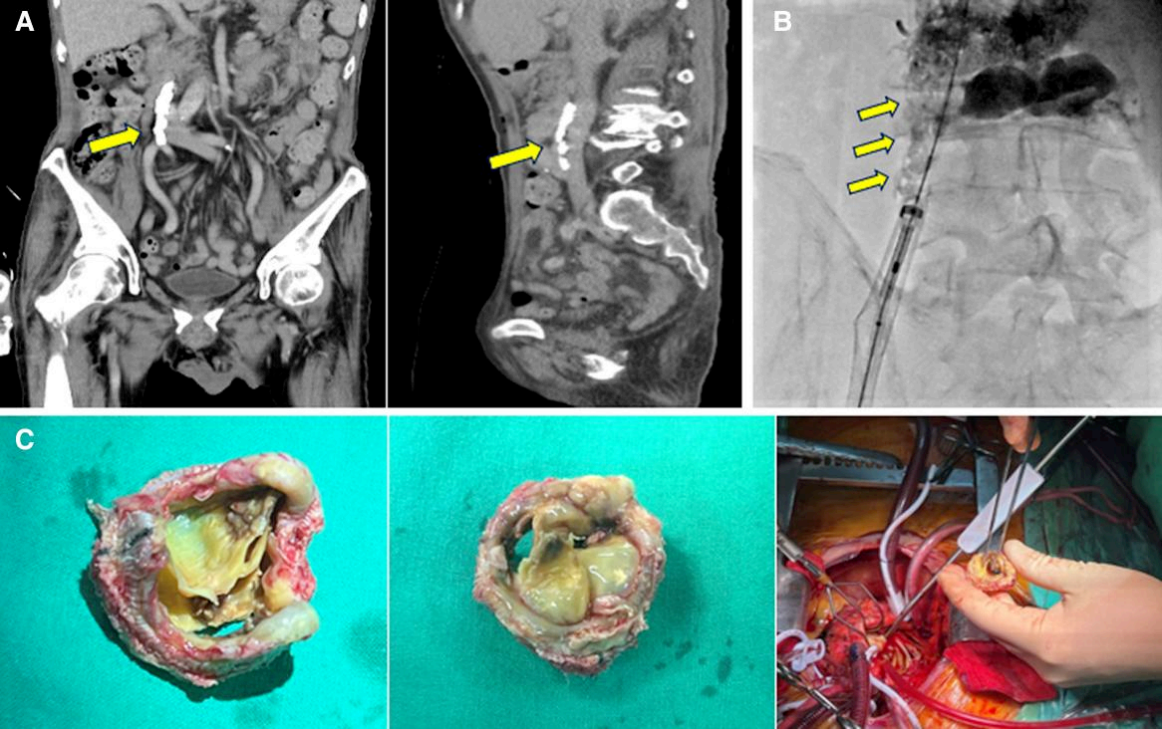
(*A*) The CT scan before transcatheter procedure showed extensive calcification. (*B*) Venous calcification appearing as a ‘broken eggshell’ pattern on fluoroscopic imaging. (*C*) Failed mitral bioprosthesis with pliable anterior leaflet and focal calcification with margin tearing of posterior leaflet.
